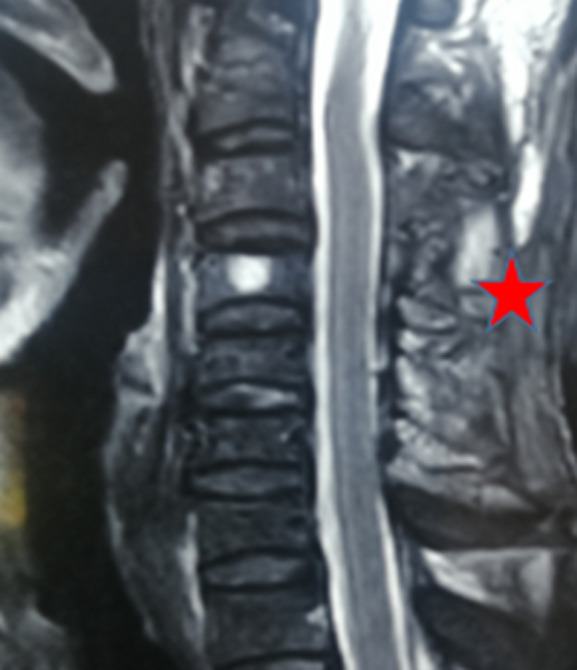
magnetic resonance imaging sagittal view postoperative follow-up showing C3-C5 laminectomy (red stars) without hematoma
